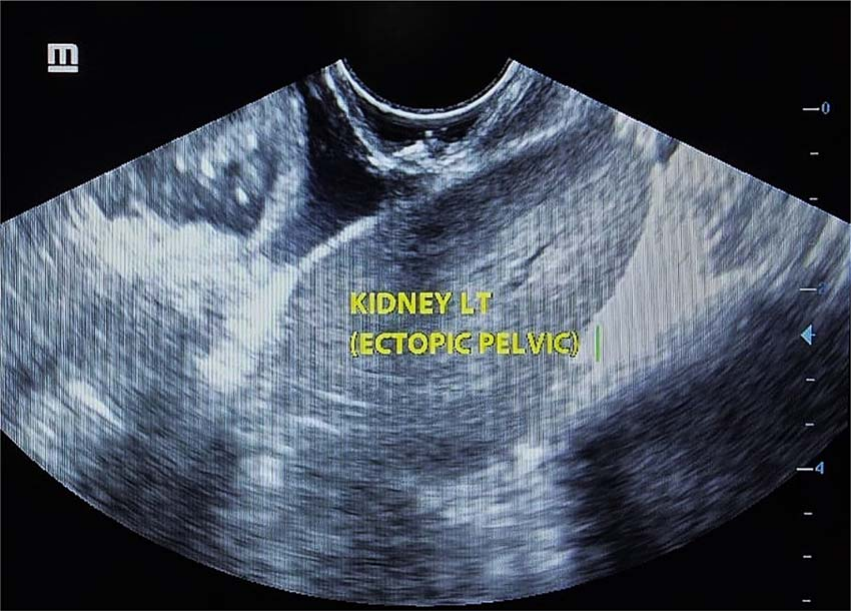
Ultrasound of abdomen showing left ectopic pelvic kidney.
